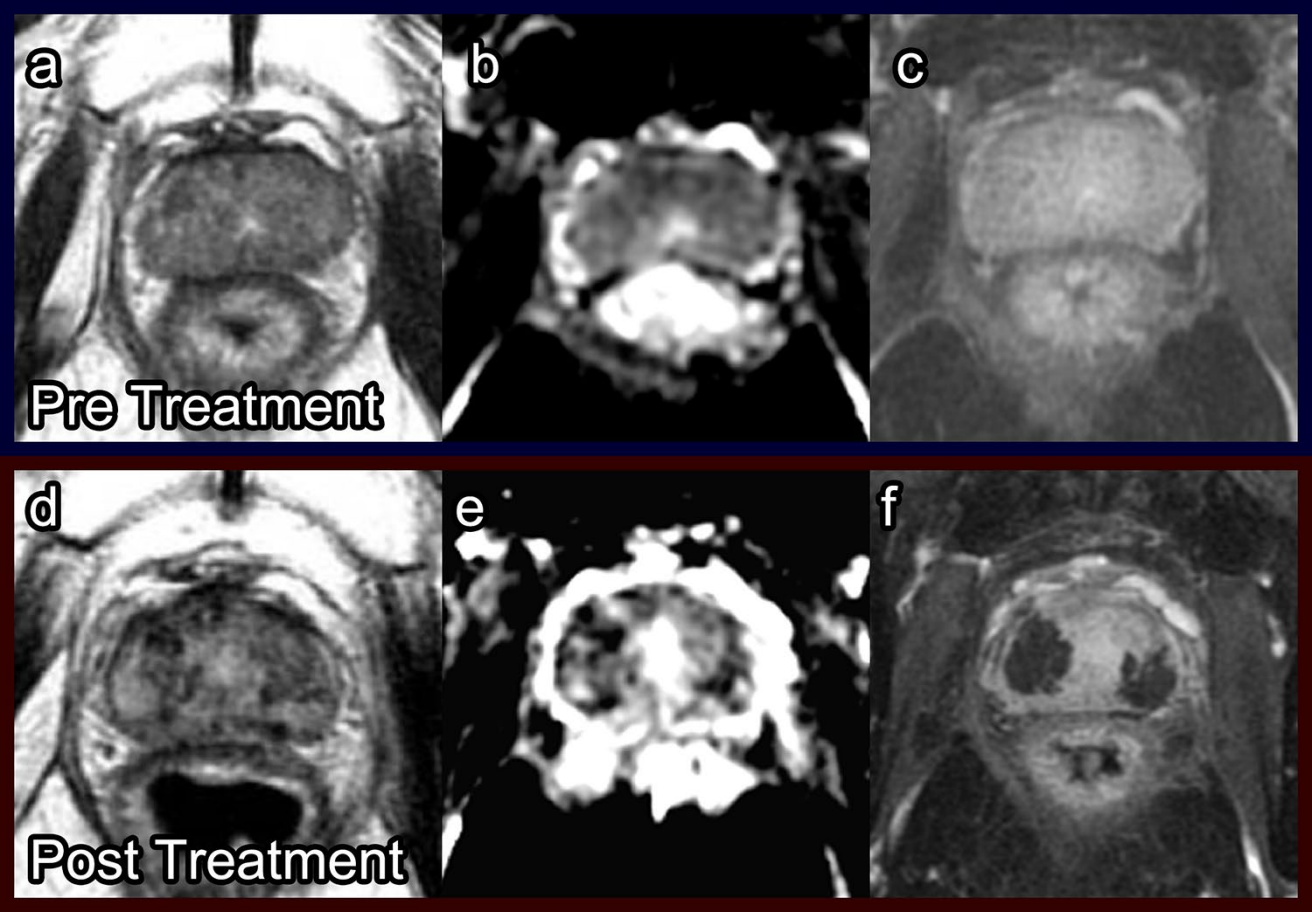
Photodynamic therapy. Pre-treatment prostate MRI in a patient with history of external beam radiation therapy for prostate cancer: T2 (a), ADC (b), and DCE (c) demonstrate heterogeneous prostate enhancement, diffuse restricted diffusion, and heterogeneous T2 dark signal. Post-photodynamic therapy prostate MRI at 7 days: T2 (d), ADC (e), and DCE (f) show post-treatment effect best on the post-contrast imaging with areas of nonenhancement
